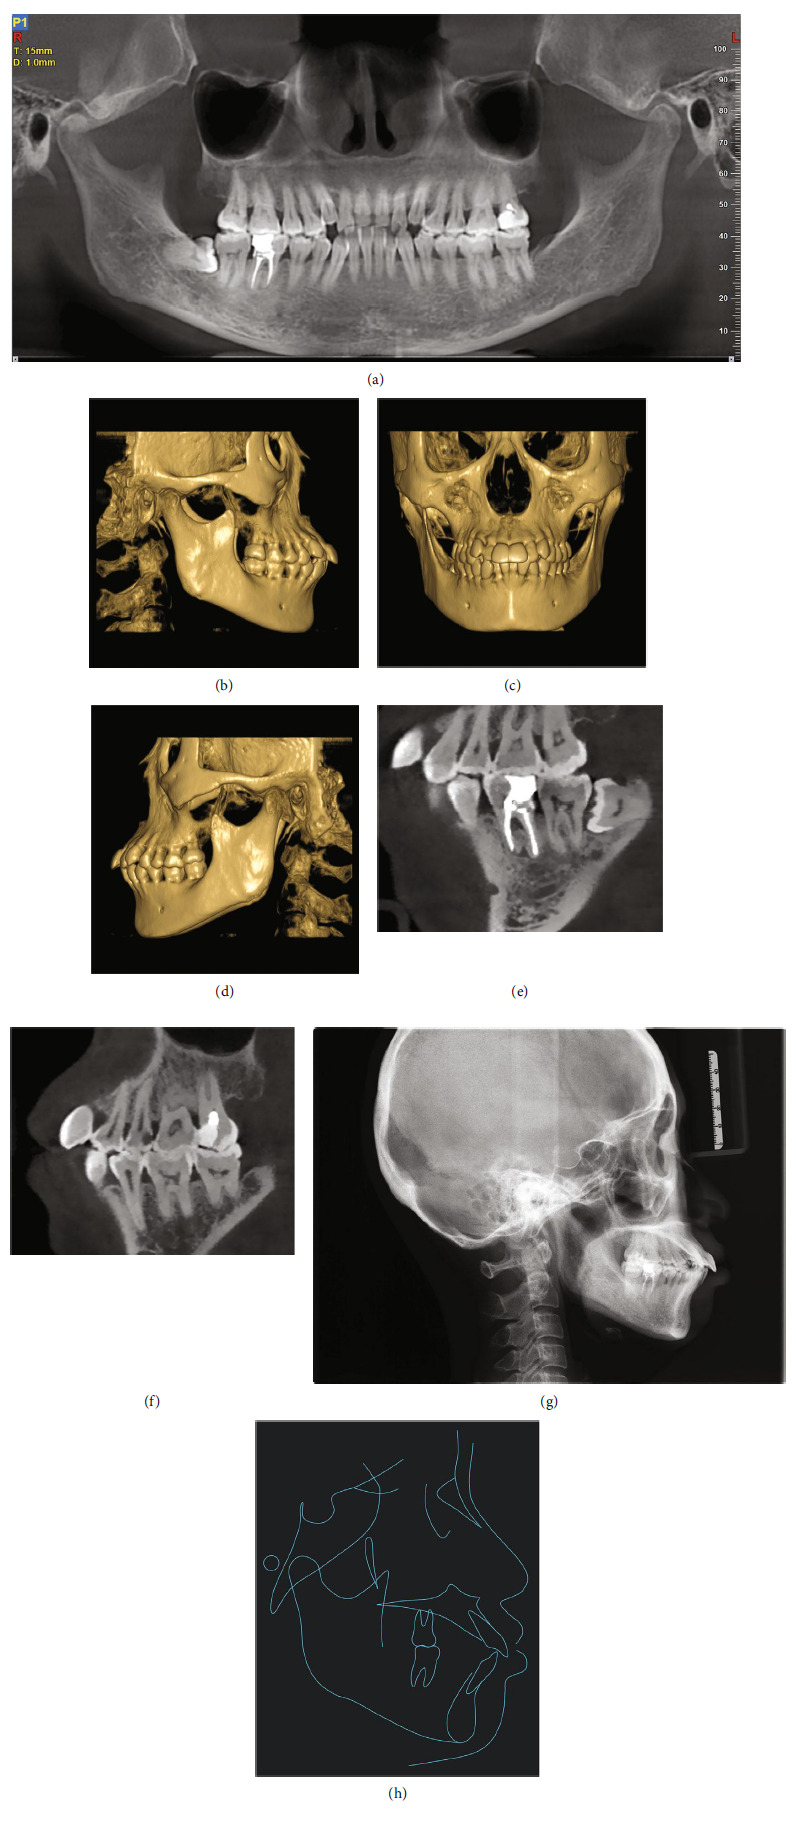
Pretreatment radiographs. (a) Panoramic radiograph sectioned from CBCT. (b–d) Three-dimensional reconstruction of CBCT. (e) The mandibular right first molar exhibited obvious radiolucency in the furcation and apical region, with root resorption. (f) The maxillary left second premolar exhibited a radiolucency in the distal regions of the crown. (g) Lateral cephalogram. (h) Lateral cephalometric tracing.
